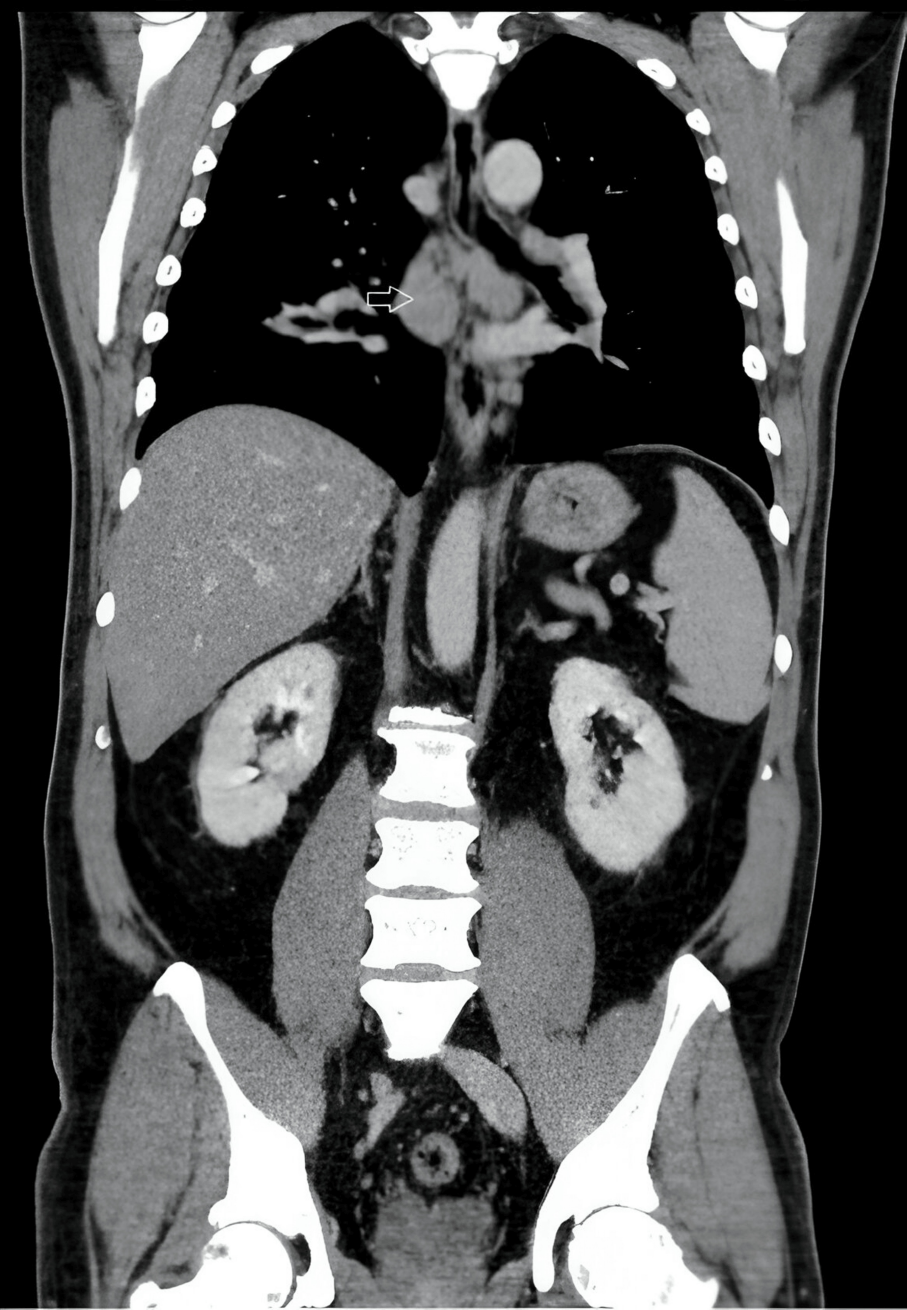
CT scan revealing multiple mediastinal and bilateral hilar adenopathies, the largest measuring approximately 28 mm
